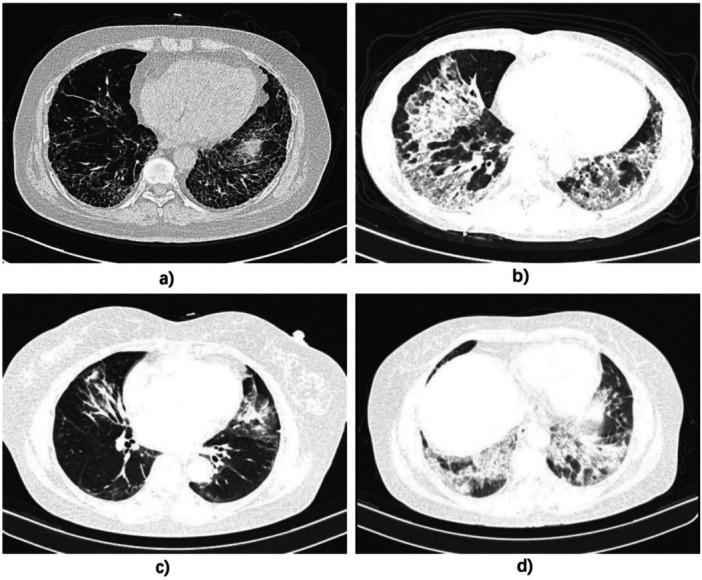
Typical HRCT images for ASS‐ILD patients. (a) HRCT image in an anti‐EJ antibody‐positive ASS‐ILD patient, which exhibited UIP pattern and the patient experienced recurrence at 18 months after diagnosis. (b) HRCT image in an anti‐Jo‐1 antibody‐positive ASS‐ILD patient, which exhibited NSIP with OP pattern and the patient has not experienced recurrence. (c) HRCT image in an anti‐Jo‐1 antibody‐positive ASS‐ILD patient, which exhibited NSIP pattern and the patient experienced recurrence at 8 months after diagnosis. (d) HRCT image in an anti‐Jo‐1 antibody‐positive ASS‐ILD patient, which exhibited OP pattern and the patient has not experienced recurrence.
